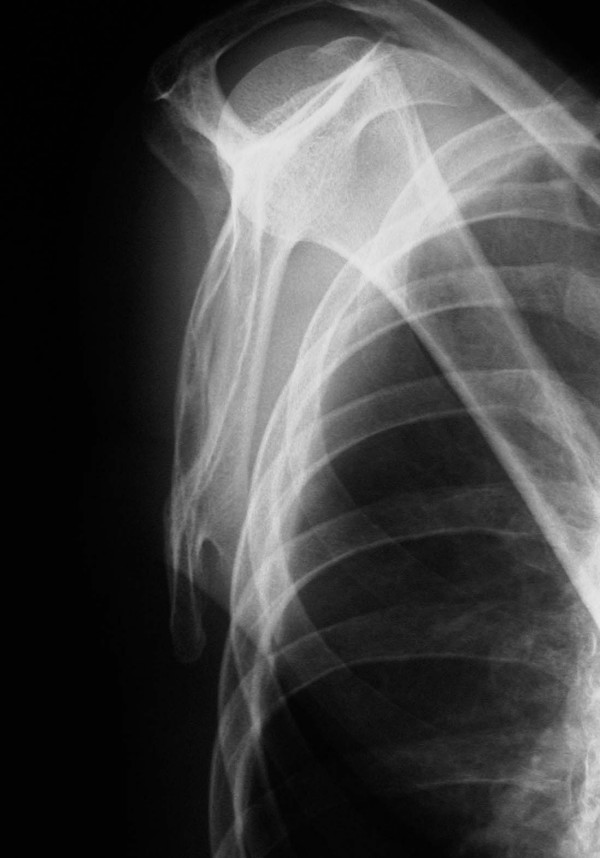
A trans-scapular roentgenogram shows the pedunculated shape of an osteochondorma at the inferior aspect of the anterior scapula.
